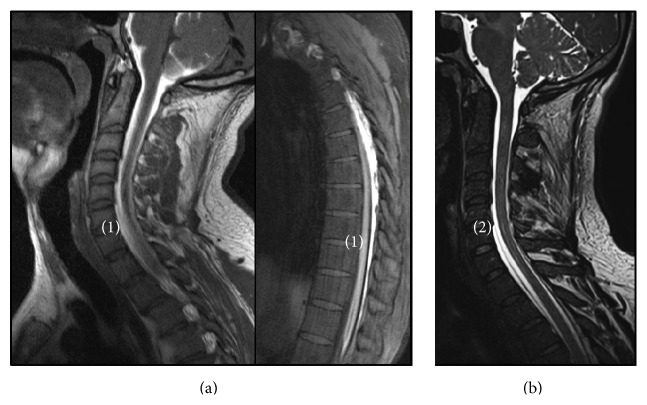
(a) T1-weighted spine MRI scan after intrathecal administration of gadolinium: sagittal views showing extra-arachnoid cerebrospinal fluid collections enhanced with gadolinium (1). (b) T2-weighted spine MRI scan showing subdural fluid collections and site of leak (2).
